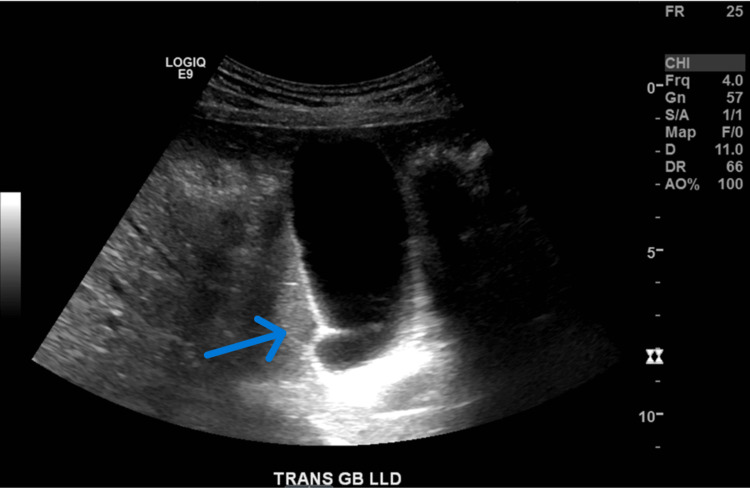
Abdominal ultrasound (US) revealing no stones and minimal gallbladder sludge
